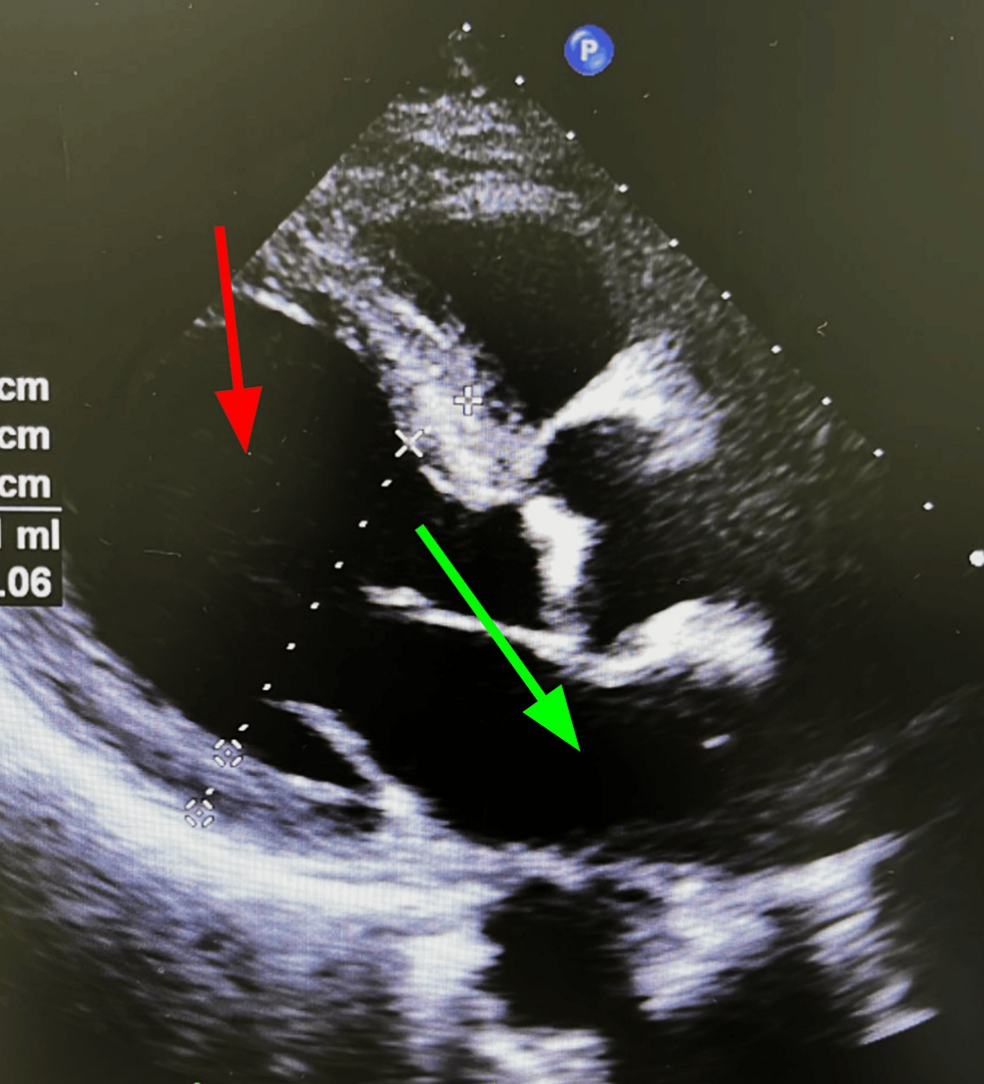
Echocardiogram with suggestive features of dilated cardiomyopathy. Left ventricular diastolic diameter of 48 mm, left ventricular systolic diameter of 40 mm, and LVEF of 45% (red arrow) and left atrial enlargement (green arrow). LVEF: left ventricle ejection fraction
